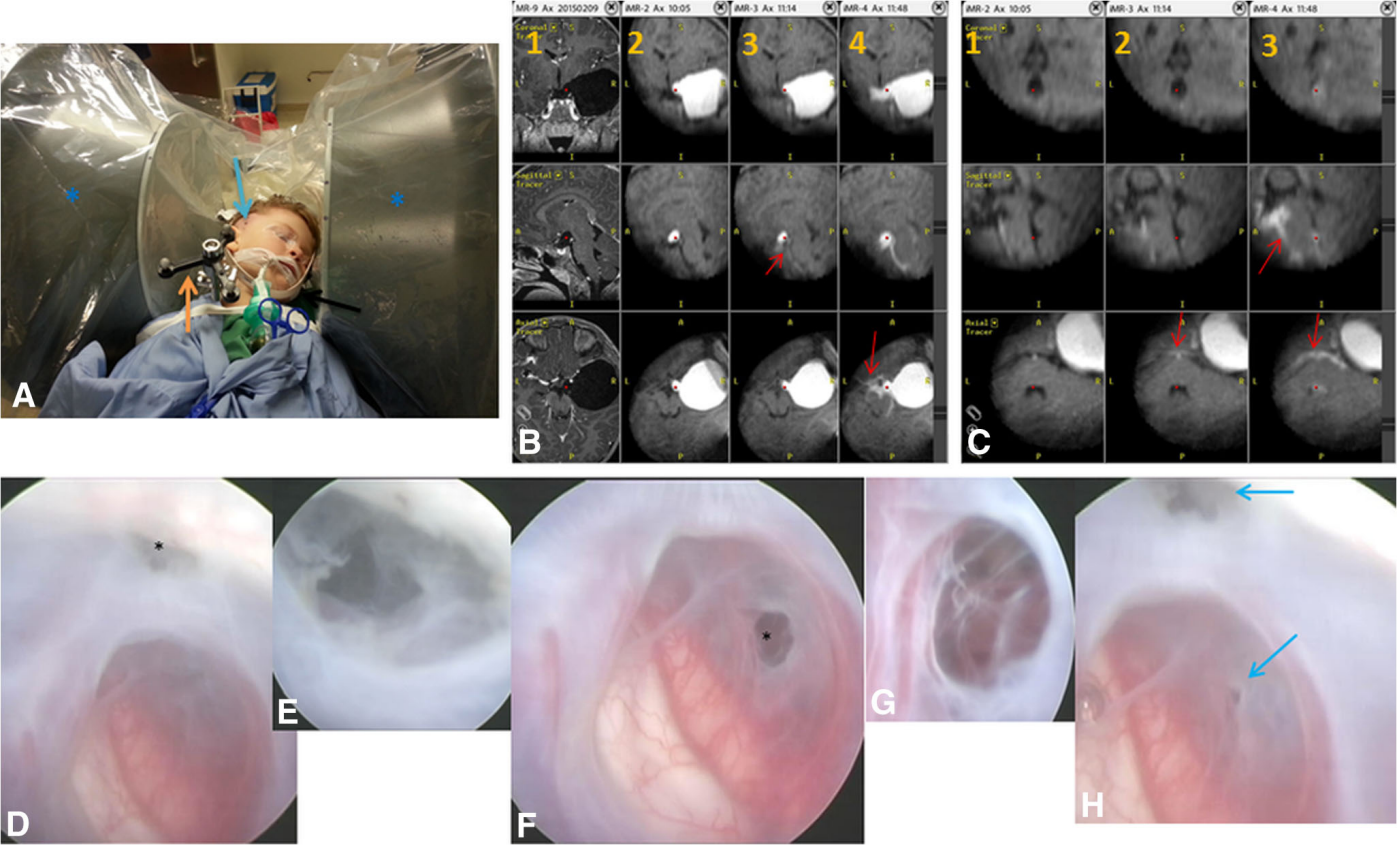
A 4-year-old girl with an arachnoid cyst of the right middle fossa (patient 10). a Photo showing the patient positioning. The head was fixed in a nonferomagnetic clamp. A receive coil was placed on the head around the operative field and connected to the low-field iMR imager (black arrow). Orange arrow is pointing the passive navigation reference frame. Blue arrow is showing on the skin the planned surgical entry point. The intraoperative MR scanner (blue asterisk) is placed in a position ready for the performance of the first positioning scan. b Column 1—preoperative high-field T1-weighted contrast enhanced scan showing the expansive character of the cyst. Columns 2, 3, and 4 show the result from low-field iMRI imaging. The contrast delivery into the cyst shows no patency of the arachnoid cyst (column 2). After initial cyst fenestration, there is only some insufficient contrast flow through the cyst (column 3, red arrow). The performance of a second larger fenestration resulted in evident contrast flow from the cyst to the neighboring arachnoid cisterns (column 4, red arrow). c Further intraoperative images comparing the prefenestration cyst appearance (column 1), the appearance after the first fenestration with minimal contrast flow (column 2, red arrow), and the improved contrast flow into more distally located arachnoid cisterns after the conduction of the second fenestration (column 3, red arrow). d Intraoperative endoscopic view of the inner part of the cyst. The asterisk points the initially performed fenestration, in proximity to the petroclinoidal fold. e Enlargement of the area of fenestration shown in d. f View of the second fenestration (asterisk) conducted after obtaining of the result from iMRI cysternography that showed insufficient contrast flow through the first stoma. The second stoma was performed between the posterior communicating and choroidal artery. g Enlargement of the area of fenestration shown in f. h View of both fenestrations (blue arrows)
